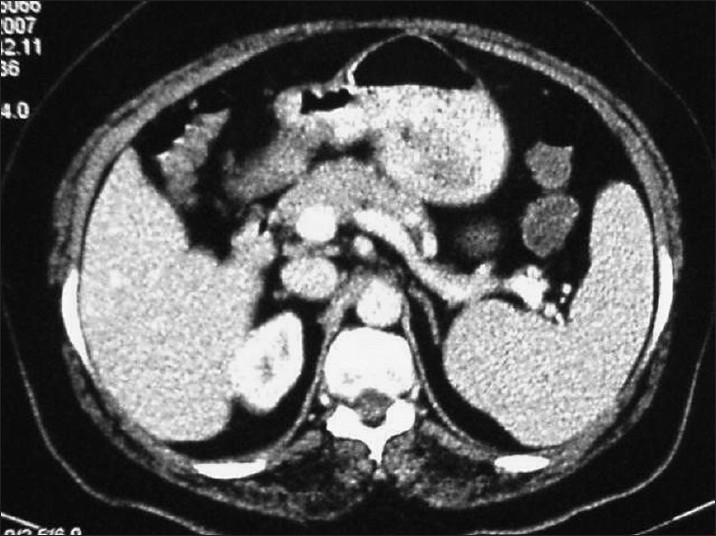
CT scan showing focal thickening of the gastric antrum.
